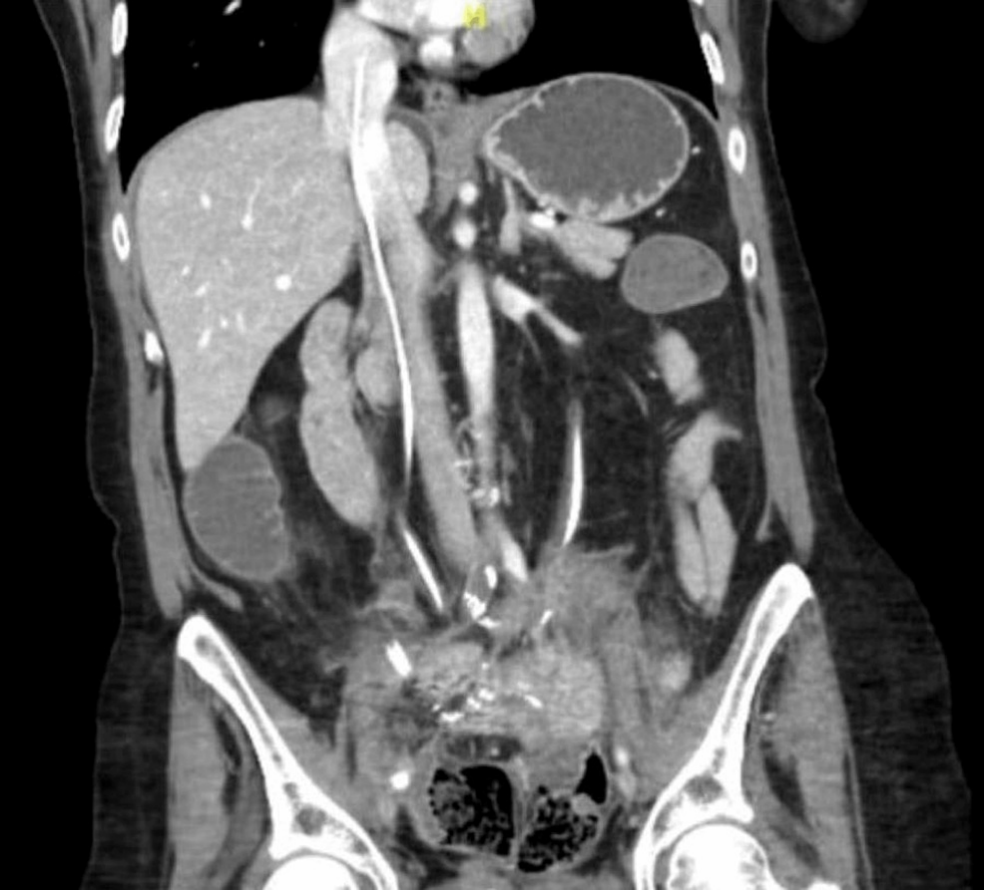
Coronal view CT abdomen and pelvis with IV contrast Demonstrating a right nephroureteral stent in the IVC and right atrium IVC: inferior vena cava
